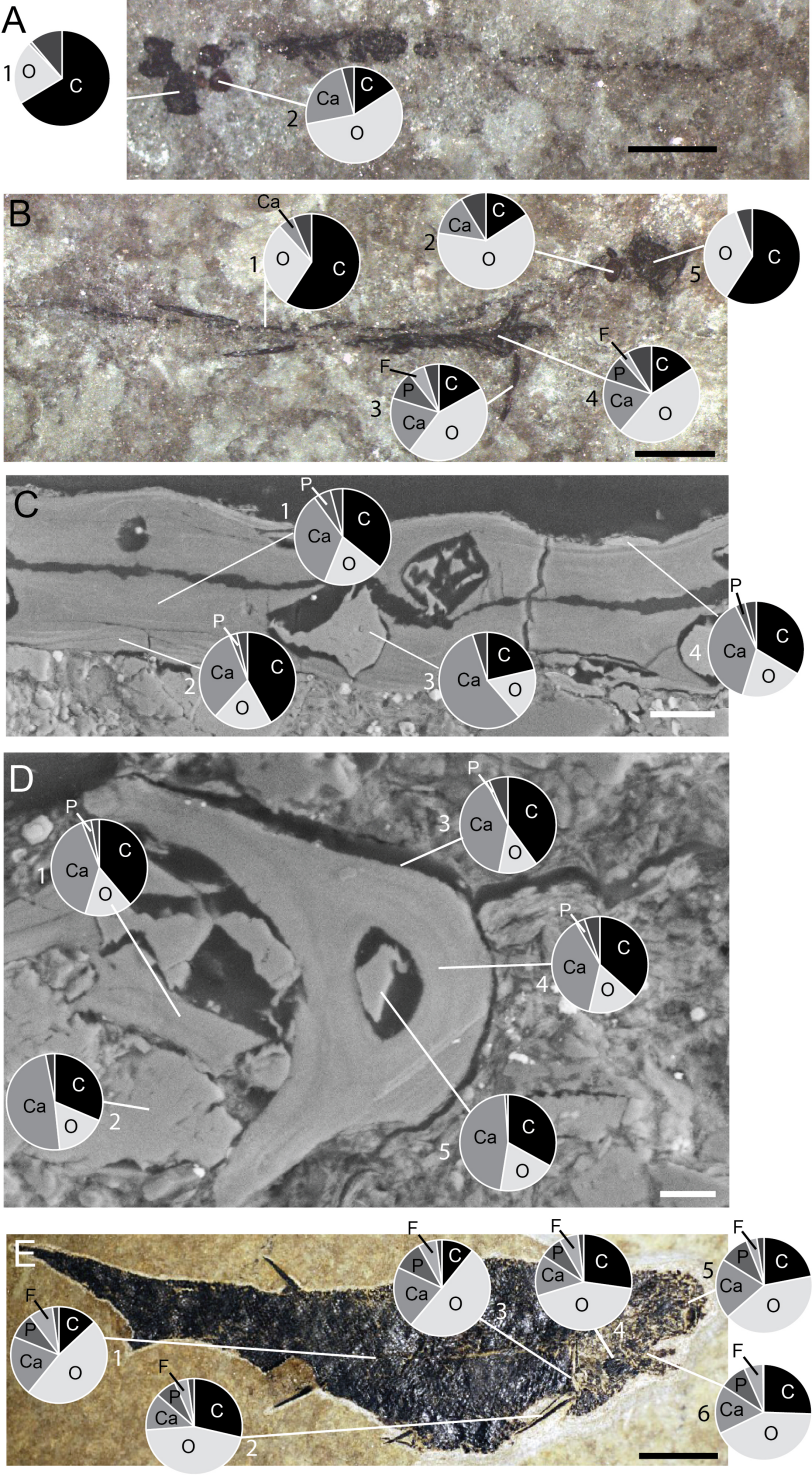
Triazeugacanthus* EDS X-ray punctual microanalyses. Pie charts represent the relative percentage of main chemical elements. (A) MHNM 03-440. Eye lenses (1) and otoliths (2). (B) MHNM 03-440. Eye lenses (5), otoliths (2), scapula (4), pectoral spine (3) and vertebral structures (1). (C) MHNM 03-398. Juvenile endoskeleton (3) and scale inner (1) and outer layers (2, 4) from transverse sections. (D) MHNM 03-398. Juvenile endoskeleton (1, 2, 5) and anal spine inner (4) and outer layers (3). (E) MHNM 03-1497. Adult sclerotic bone (5), palatoquadrate (6), branchiostegal rays (4), scapula (3), pectoral spine (2) and scales (1). Scale bars = 1 mm in (A, B); 20 µm in (C), 10 µm in (D) and 5 mm in (E). Photo credit: Marion Chevrinais.
